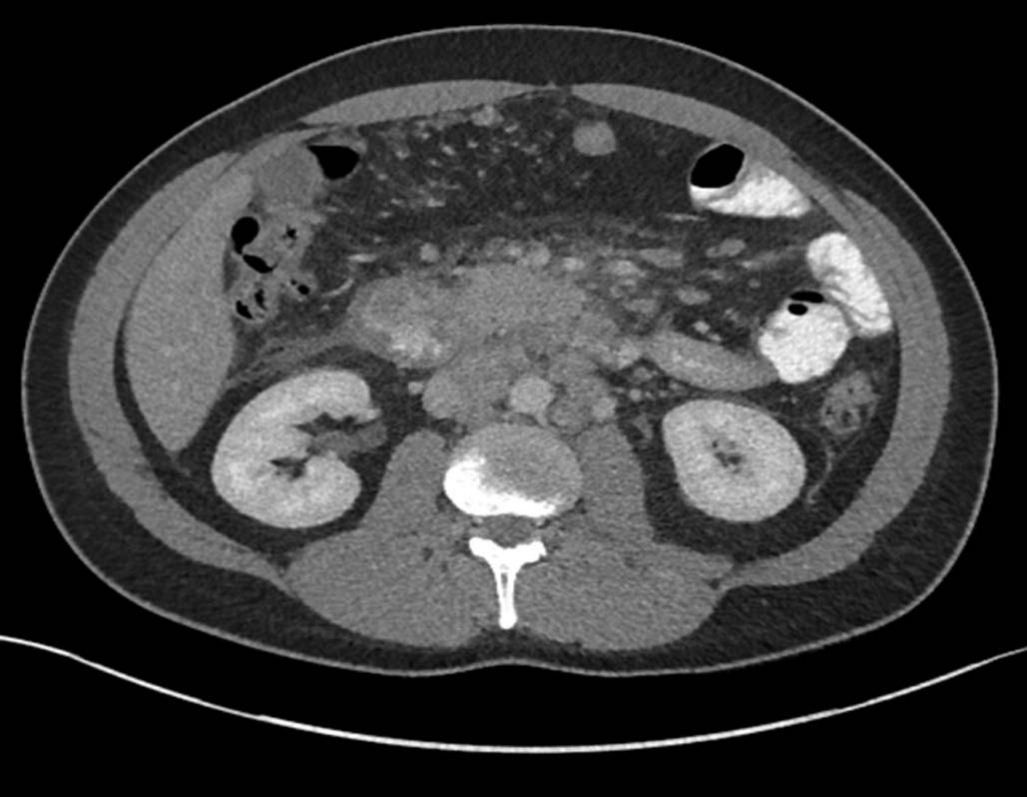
Abdominal computed tomography showing inflammation around the pancreatic head and the duodenum with retroperitoneal and peritoneal lymphadenopathy.
